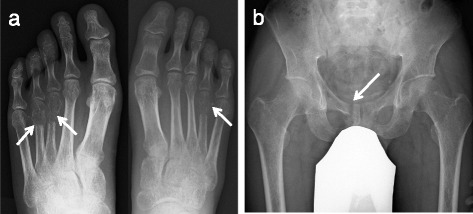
Radiographic observations. a AP radiograph shows cystic radiolucent shadow in right fourth metatarsal bone (*arrows*) and left third and fourth metatarsal bone (*arrows*). b AP radiograph shows cystic radiolucent shadow in right pubis (*arrow*)
